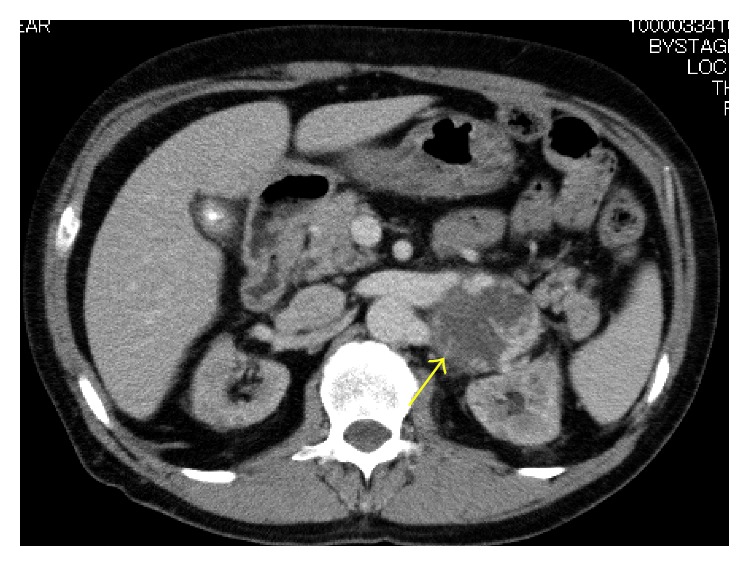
Contrast-enhanced CT. Contrast CT revealed slightly enhancement around the tumor (arrow).
